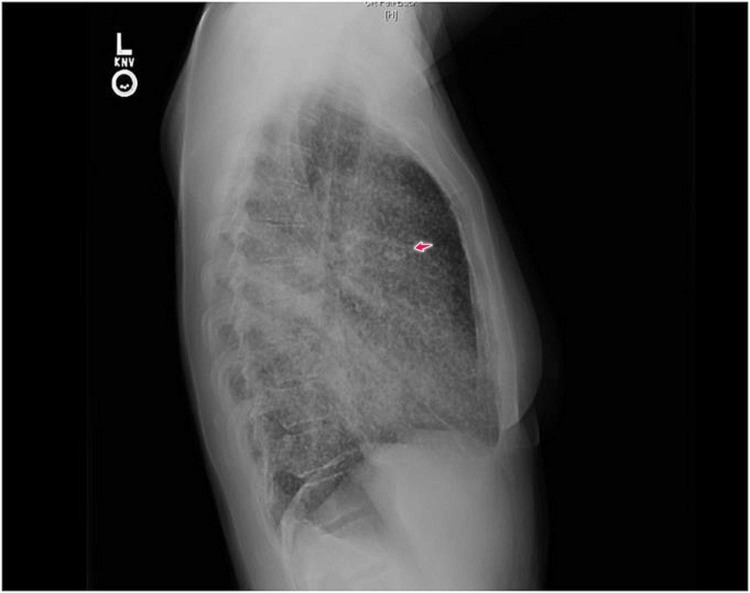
Chest X-ray, lateral view Chest X-ray, lateral view, showing extensive pulmonary infiltrates with a miliary pattern (arrow)
